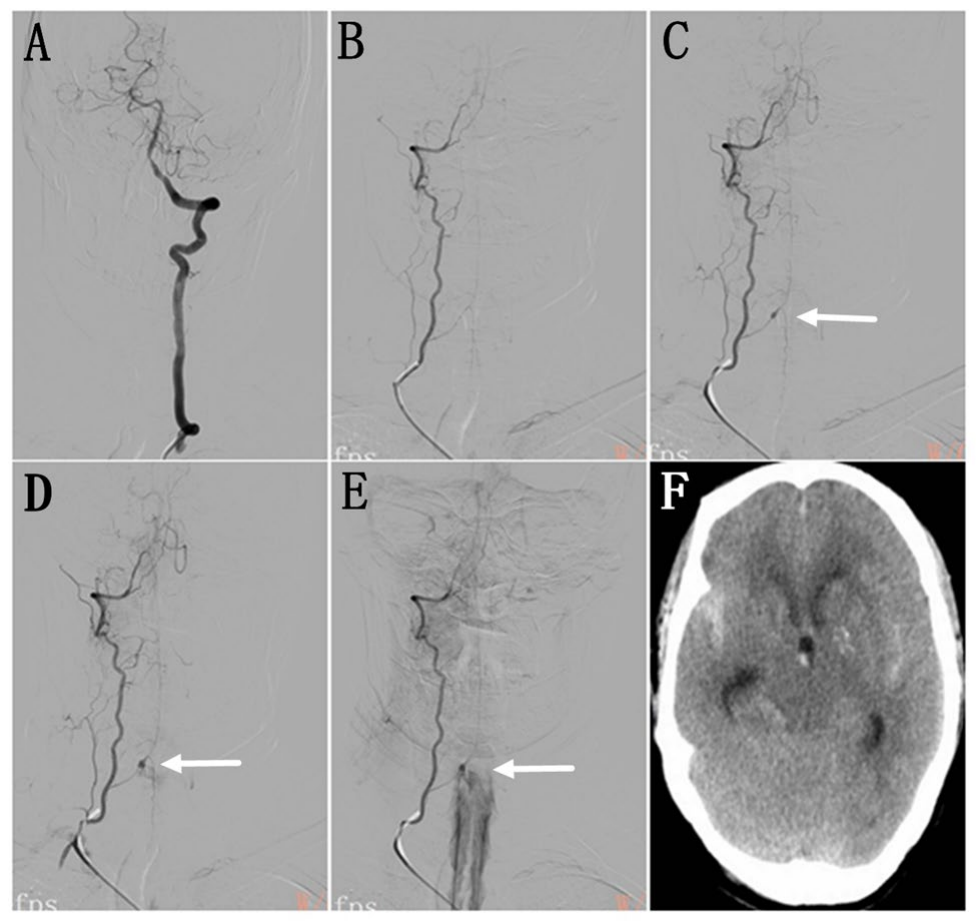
Bilateral vertebral artery angiography and Computed tomography (CT). A Left vertebral angiography showed the normal dominant vertebral artery. B Right vertebral angiography showed the hypoplastic vertebral artery and normal filling of the anterior radiculomedullary artery in the early arterial phase. C-E The subsequent angiography showed the formation and rupture of a fusiform dissecting aneurysm (arrows) in the intradural segment of the anterior radiculomedullary artery. F Head CT showed extensive subarachnoid hemorrhage
